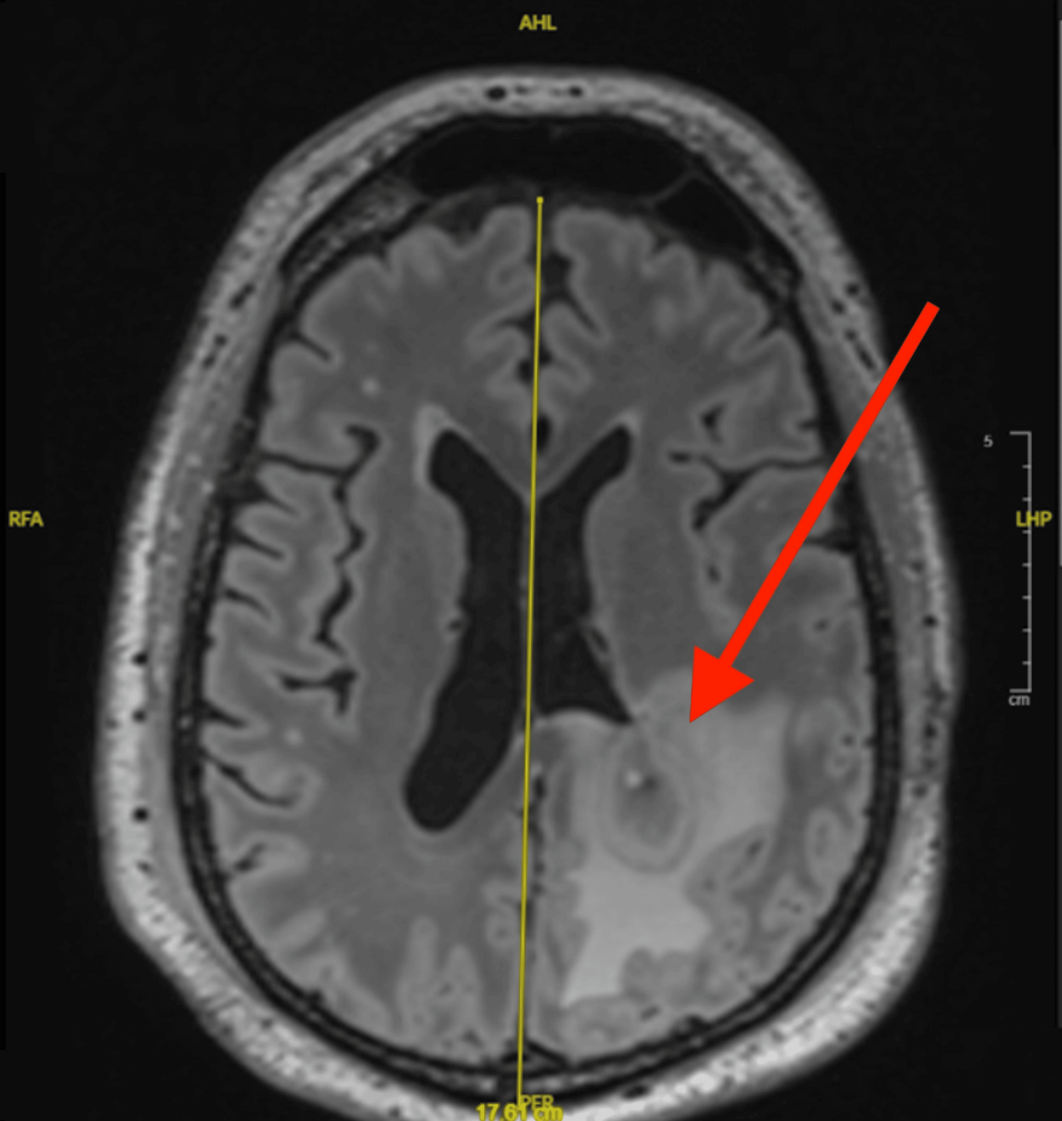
MRI with and without contrast: Axial 3D multiplanar reconstruction (MPR) FLAIR image depicting a well-defined, peripherally enhancing lesion in the left parietal subcortical white matter inferior to the resection site. The lesion shows marked restricted diffusion centrally, compatible with an abscess. MRI study dated March 13, 2024. FLAIR: Fluid-attenuated inversion recovery
